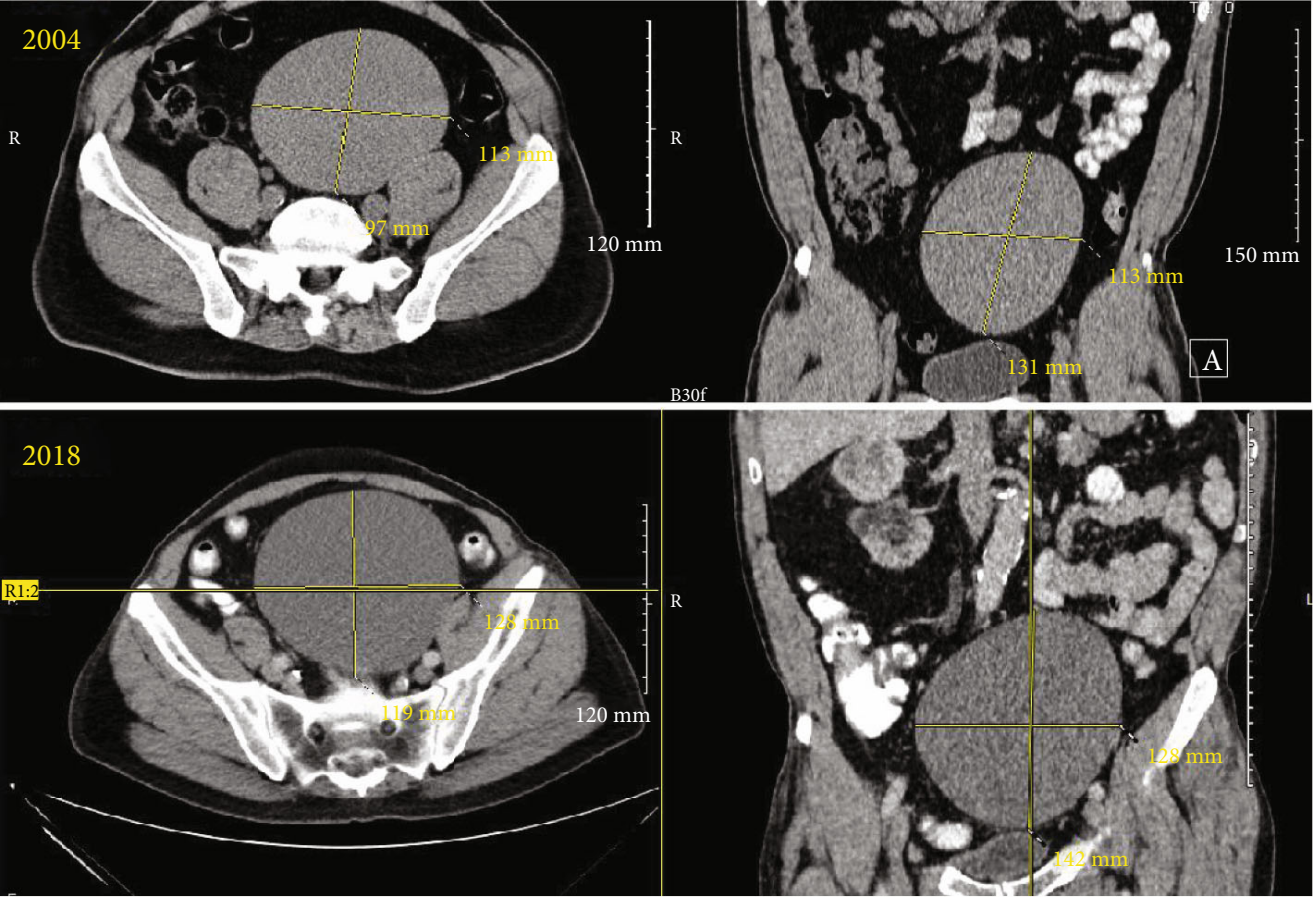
CT, axial, and coronal sections of the seminal vesicle cyst from 2004 and 2018. In 2004, the cyst measured 11.3 cm × 9.7 cm × 13.1 cm (volume approximately 500 cm^3^), while in 2018, it measured 12.8 cm × 11.9 cm × 14.2 cm (volume approximately 900 cm^3^).
